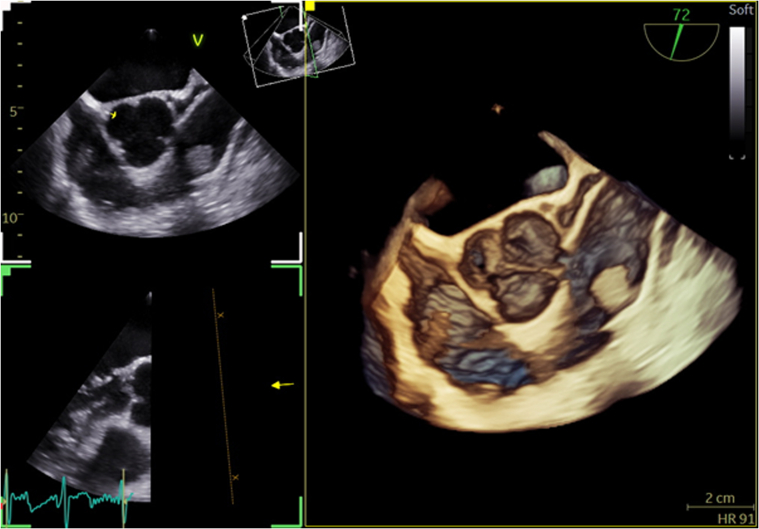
Preoperative Transesophageal Echocardiography 3D Images: Right-Sided Papillary Fibroelastoma
